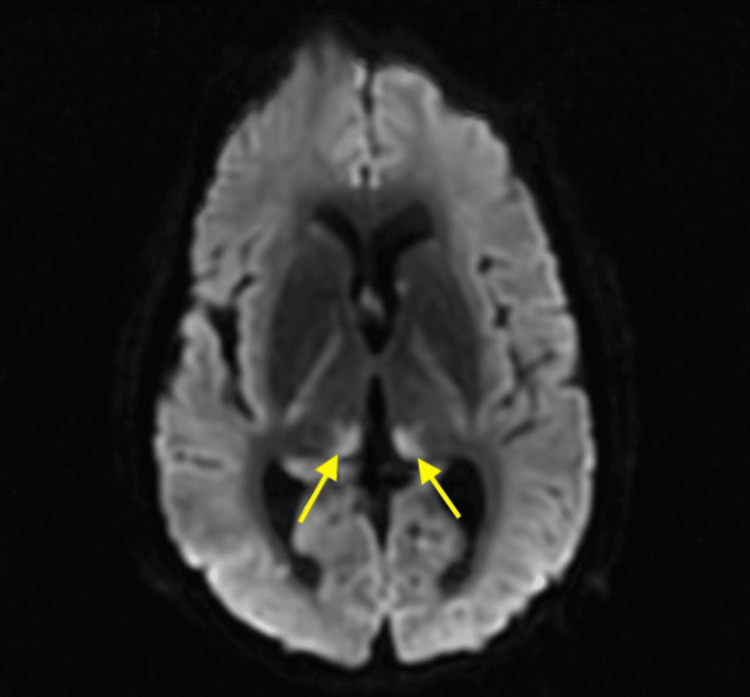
MRI diffusion-weighted imaging (DWI) of the brain revealed acute bilateral paramedian thalamic infarcts, indicated by the yellow arrows.
